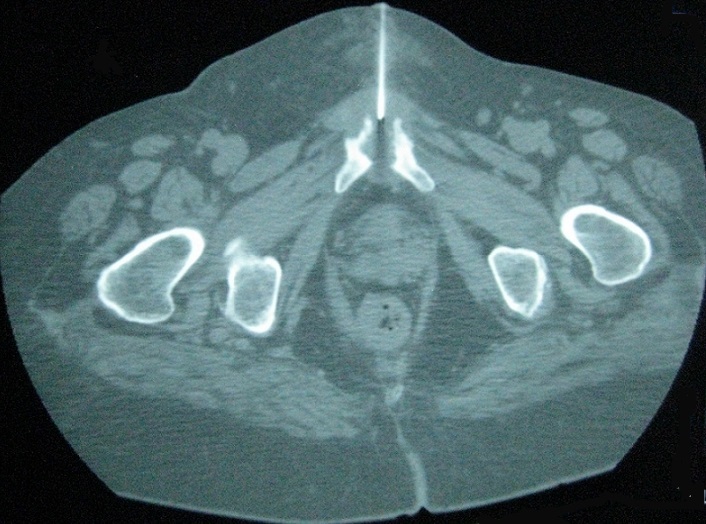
Puncture biopsy of the pubic symphysis under CT in a patient with an infectious pubic symphysitis
